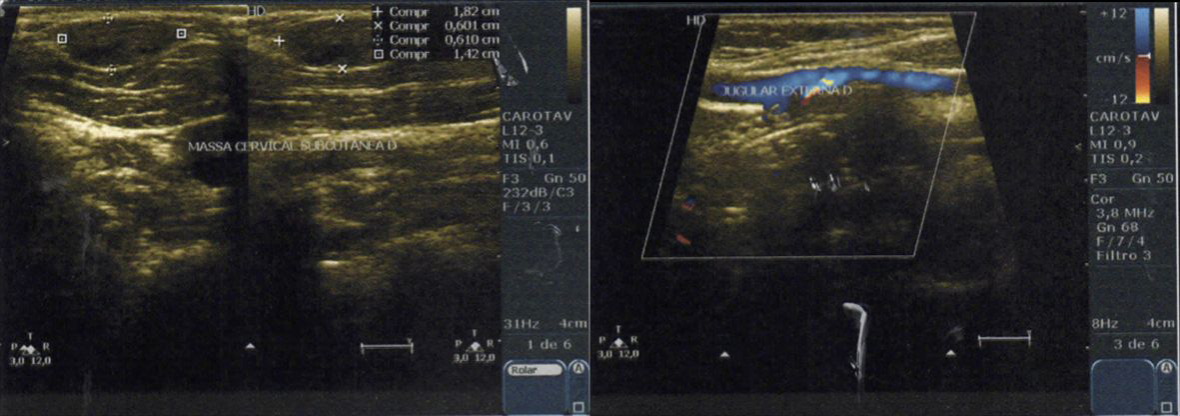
Doppler ultrasonography showing ectasia, without thrombi in the lumen and normal blood flow.
